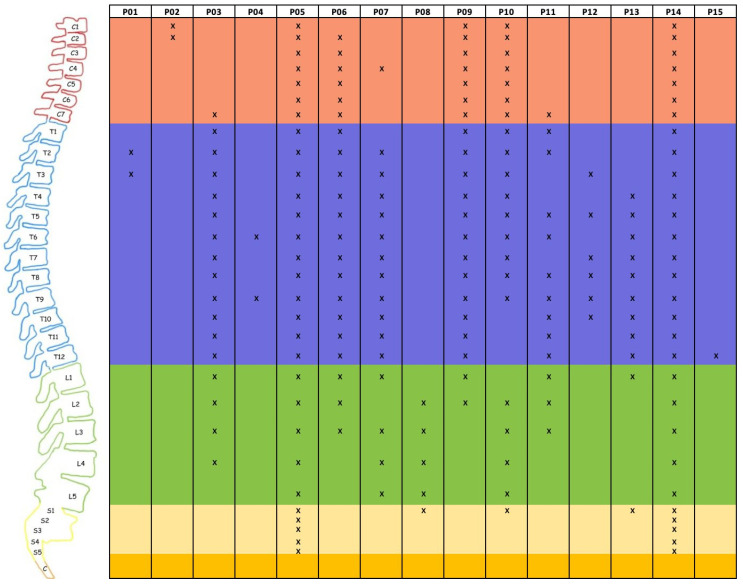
Distribution and extent of glioblastoma metastatic spinal lesions. (“X” denotes the levels of spinal cord involvement as detected on spinal MRI).
